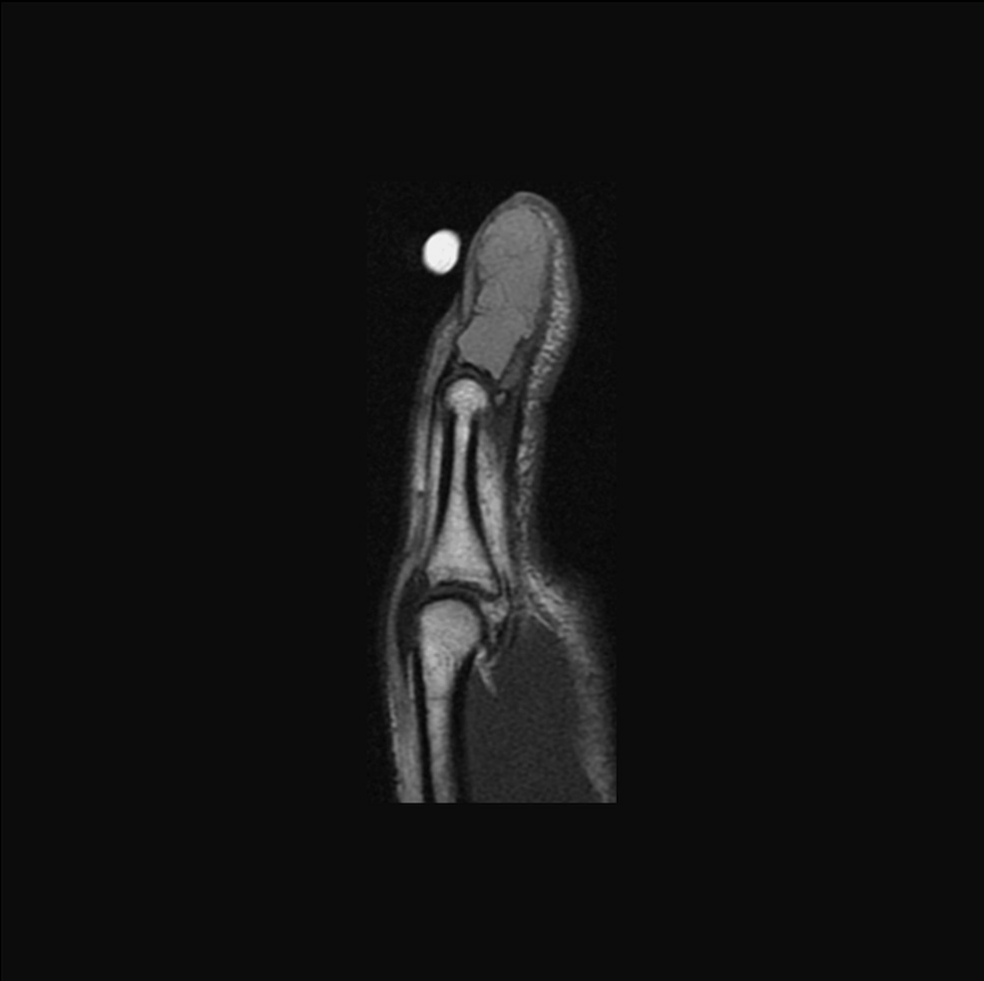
Preoperative sagittal T1 MRI of the left thumb The image is showing an expansile, multiloculated, cystic lesion of the thumb distal phalanx.
